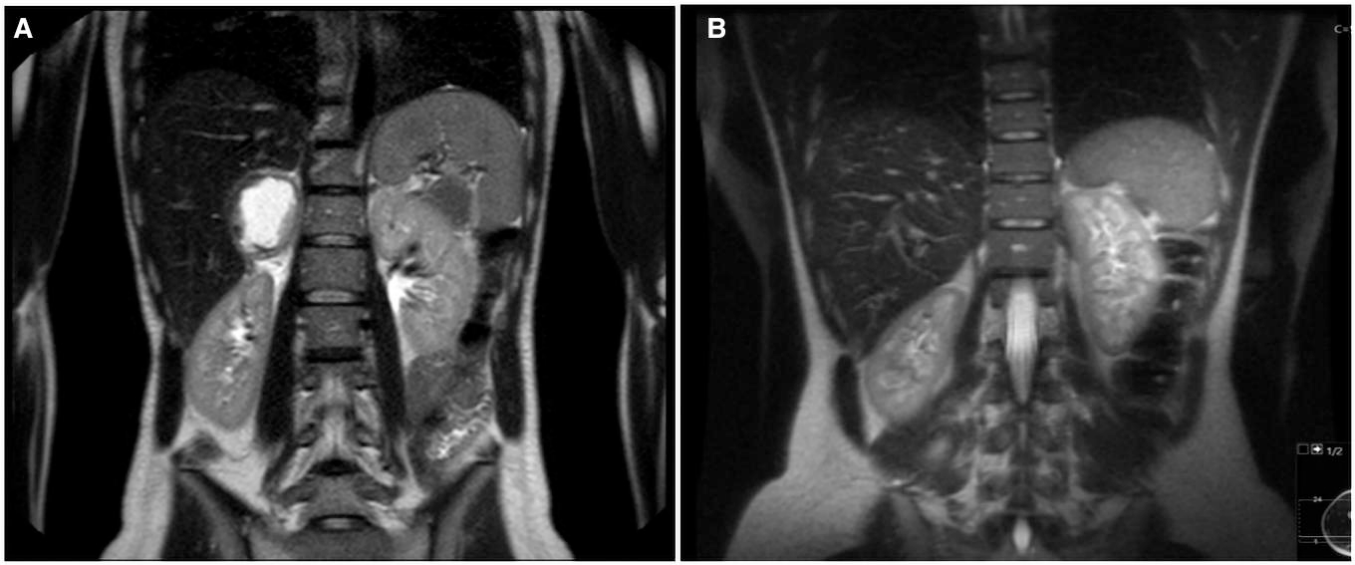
Pre-operative magnetic resonance imaging showing a right adrenal pheochromocytoma (arrow) (*A*) and follow-up imaging (*B*) showing absence of the tumour.
